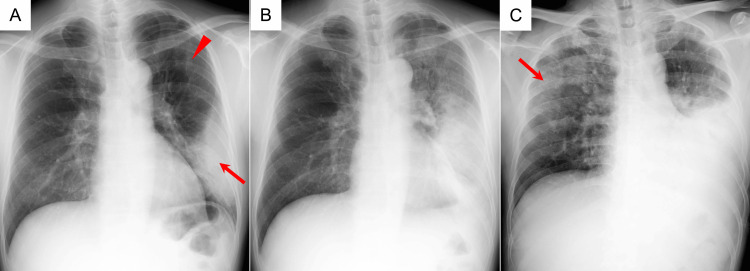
Chest X-ray findings during hospitalization (A, B, C) Chest X-ray on day 0 (admission), day 2, and day 6, respectively. (A) Wedge-shaped infiltration in the left lower lobe adjacent to the pleura (arrow), along with GGOs in the left upper lobe (arrowhead). (B) The consolidation and GGOs expand to involve the entire left lung. (C) Collapse of the left lower lobe and extension of GGOs into the right lung (arrow). GGOs, ground-glass opacities
